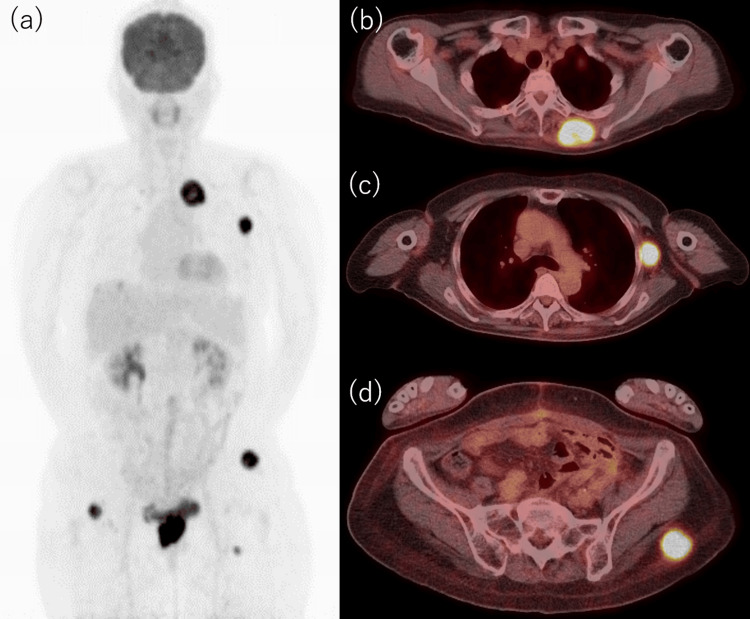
Postoperative PET-CT imaging one month after primary surgery (a) FDG uptake is observed diffusely throughout the body. (b) FDG uptake is noted in the left posterior thoracic region. (c) FDG uptake is identified in the left axillary region. (d) FDG uptake is seen in the left buttock region. All subcutaneous metastases were palpable upon physical examination FDG: 18F-fluorodeoxyglucose; PET-CT: positron emission tomography-computed tomography
